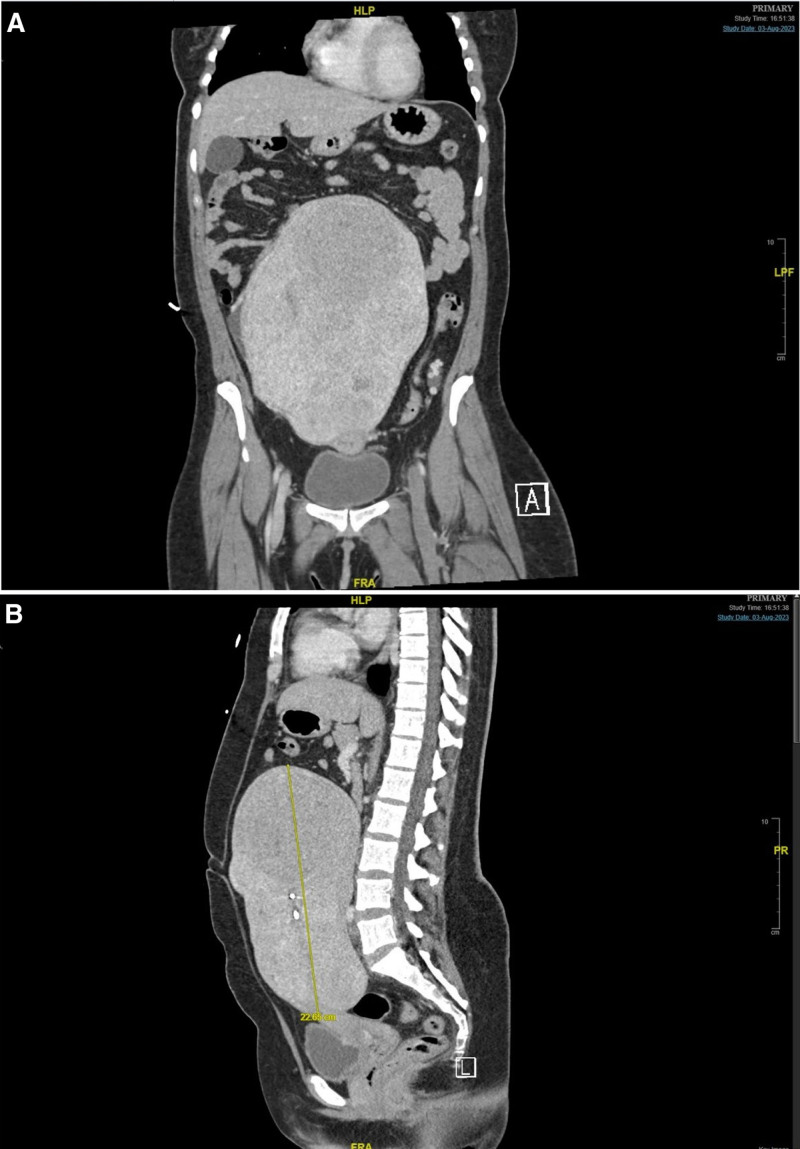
Abdominal and pelvic CT scans with contrast showing a large fibroid compressing the veins. (A) Coronal view; (B) sagittal view. CT = computed tomography.
